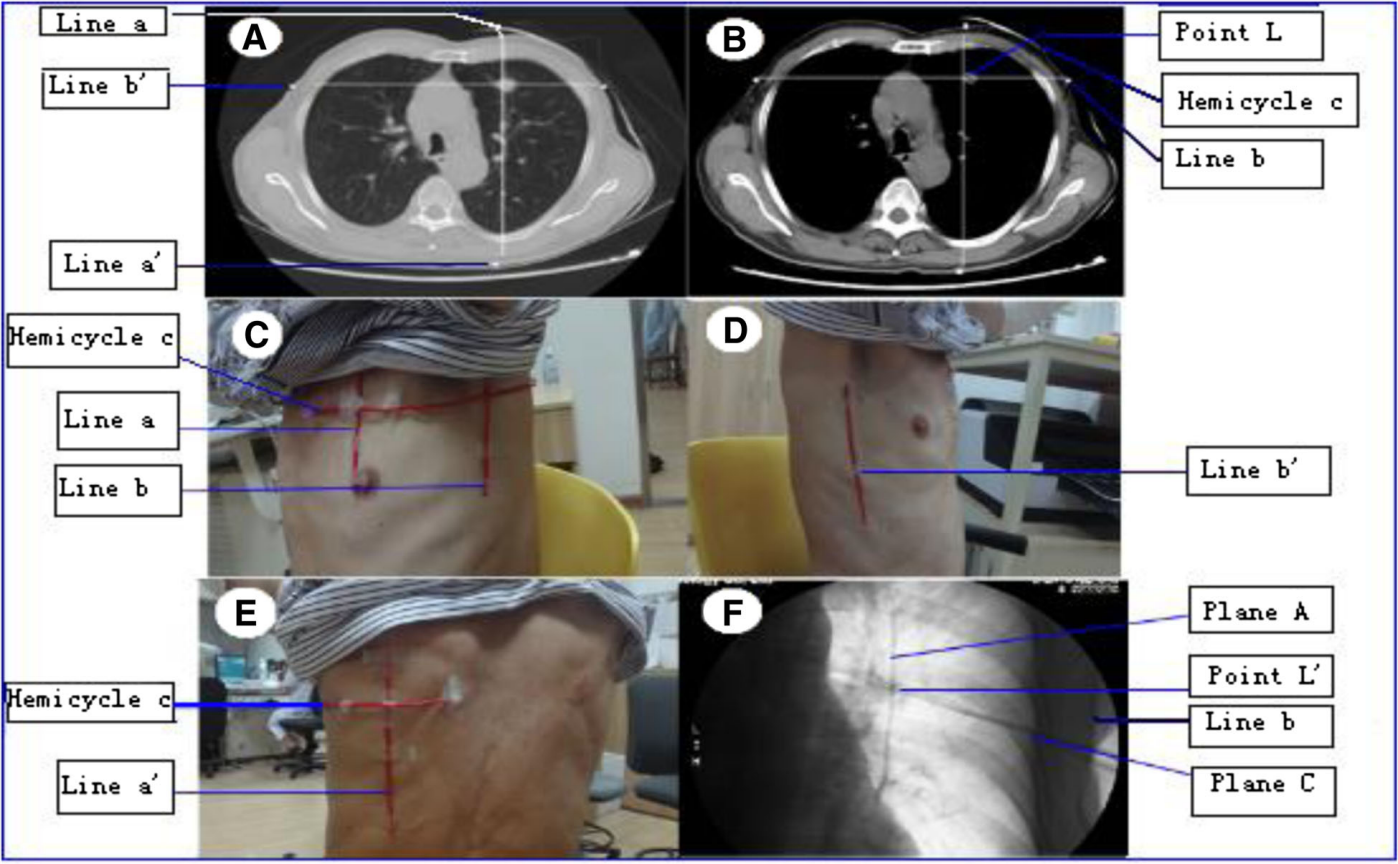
A core point of the lesion which we named as *point L* could be determined according to the crosspoint of the longest diameter of three dimensions, that is length, width and height of the lesion, on CT scans (shown by a and b). Opaque soft copper wires were respectively placed firmly on the surface of the chest wall with adhesive tape (shown by c, d and e). Under the fluoroscopy, with the careful rotation of the C-arm machine, the crosspoint *L*’ of the three perpendicular planes determined by the three sets copper wires *aa*’, *bb*’ and hemicycle *line c* (shown by a and b with *bright pots* and *lines*, and by f with *dark lines*) on the surface of chest wall could reflect and fit accurately with the *Point L* of the lung lesion (shown by f)
